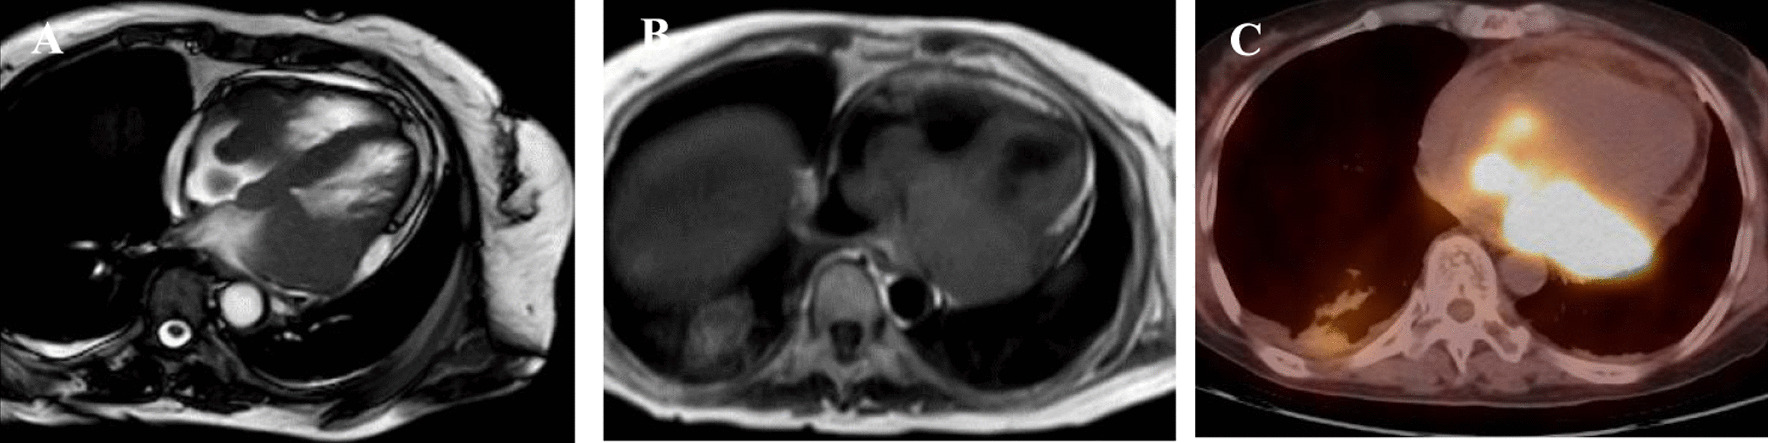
CMR confirmed left atrial mass invaded right atrium (a, b) and PET-CT scan revealed high FDG uptake in atrum and mediastinal lymph nodes (c)
